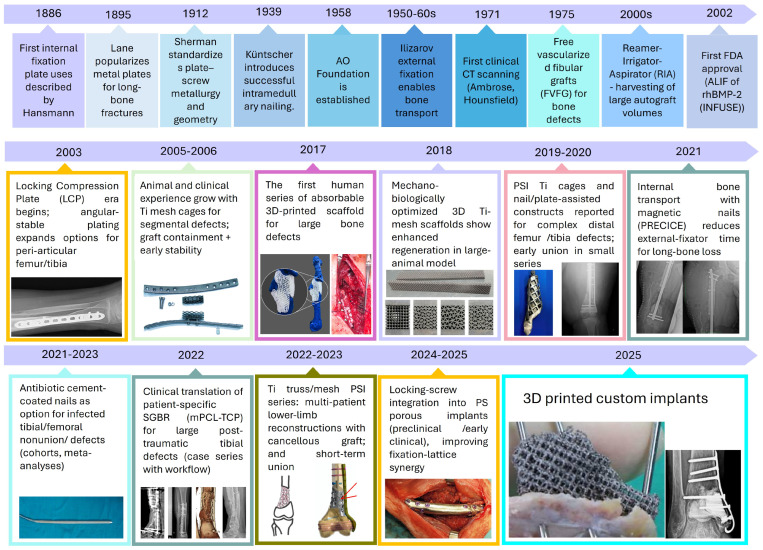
Historical evolution of reconstruction strategies for segmental femoral and tibial defects (1886–2025). The timeline from early internal fixation plates, intramedullary nailing, Ilizarov external fixation, and CT-based planning through to locking compression plates, titanium mesh cages, magnetic lengthening nails, and contemporary patient-specific solutions such as Ti lattice cages, mPCL-TCP SGBR scaffolds, and custom 3D-printed implants [3,51,52,53,54,55,56,57,58,59,60,61].
